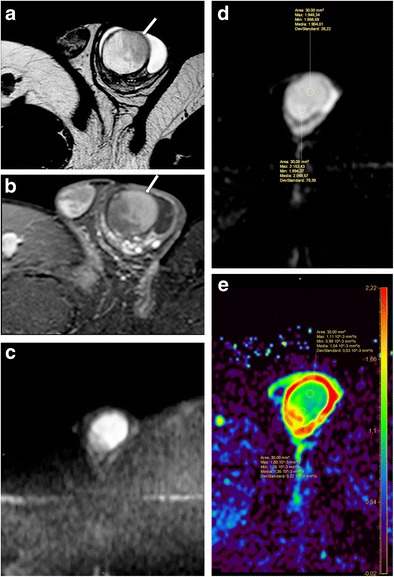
a-e MR characterization of post-traumatic fibrosis. a T2-weighted TSE sequence, axial view and b post-contrast T1-weighted SPIR TSE sequence, axial view, depicting an ill-defined hypointense on T2-weighted image (T2-wi) area, with mild contrast enhancement (arrow), consistent with fibrosis; c DWI, b = 800; d-e ADC map
